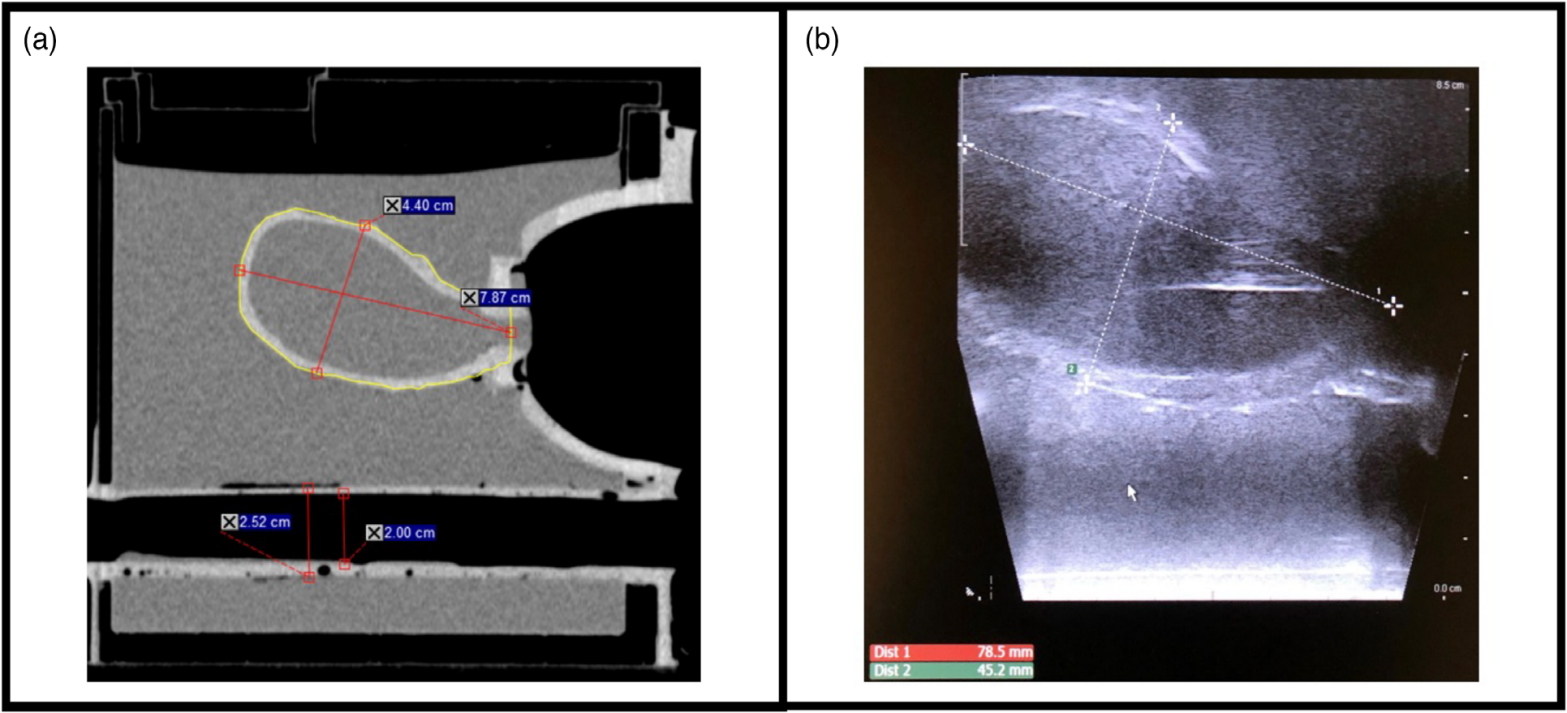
(a) Sagittal CT image showing measured uterus (length x width) and rectum; (b) Sagittal US image with uterus dimensions.
